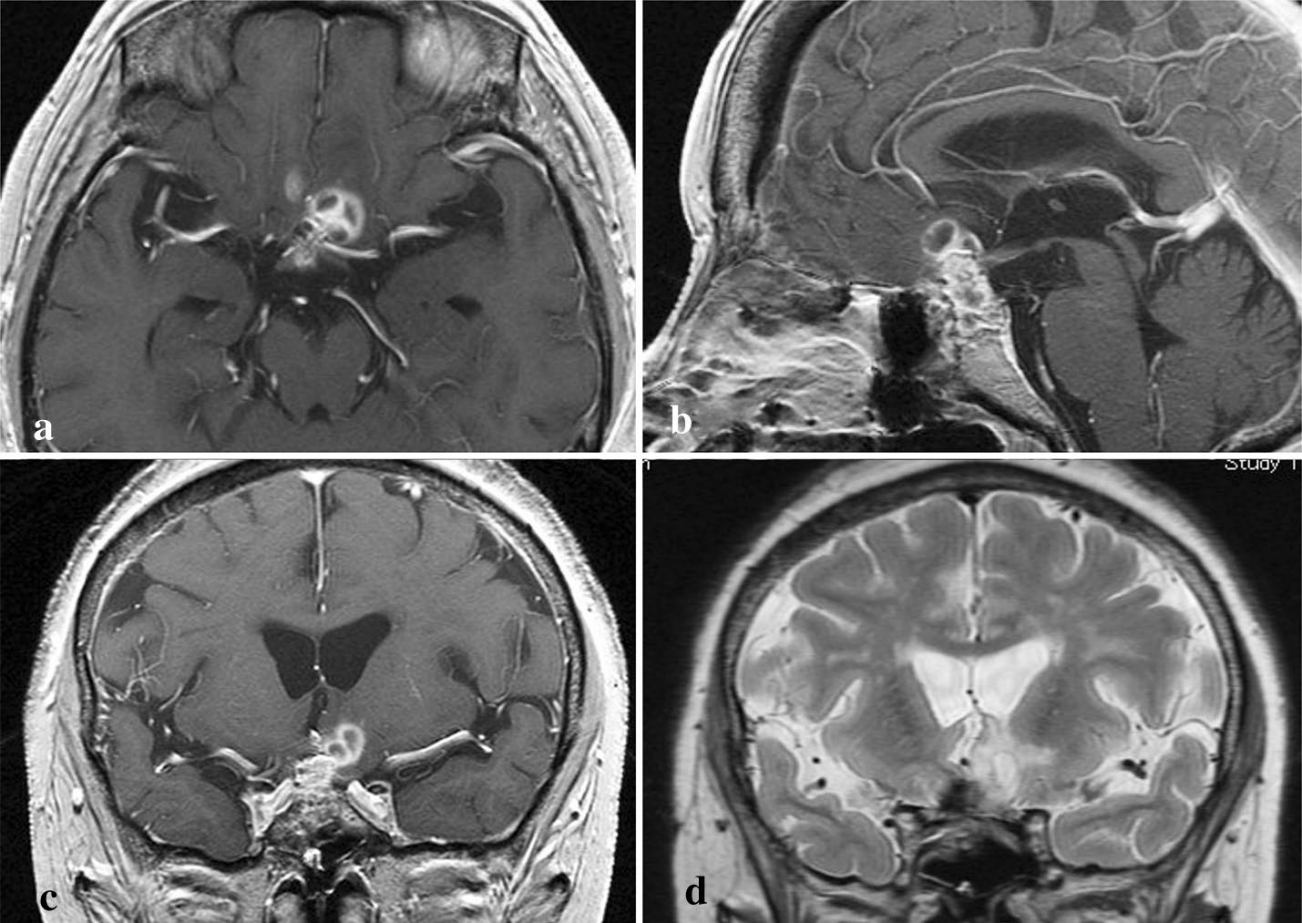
Case 2. Preoperative axial (a) and sagittal (b) T1-weighted MR images with gadolinium revealing a multicystic suprasellar lesion infiltrating into the bilateral frontal lobes. c Preoperative coronal T1-weighted MR image with gadolinium revealing infiltration of the lesion into the left rectal gyrus. d Preoperative coronal T2-weighted MR image revealing an extensive irregular high intensity area in the left frontal lobe
